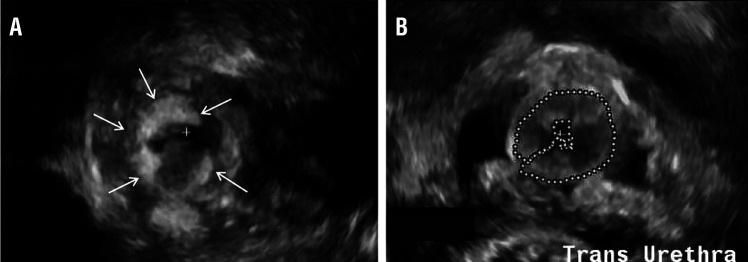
(+)=Urethral lumen; 3DUS=Three-dimensional ultrasound. 3DUS reveals asymmetric MPQ configuration (A) compared to a symmetrical “horseshoe configuration” (B). MPQ is indicated by either arrows (A) or as outlined (B).
